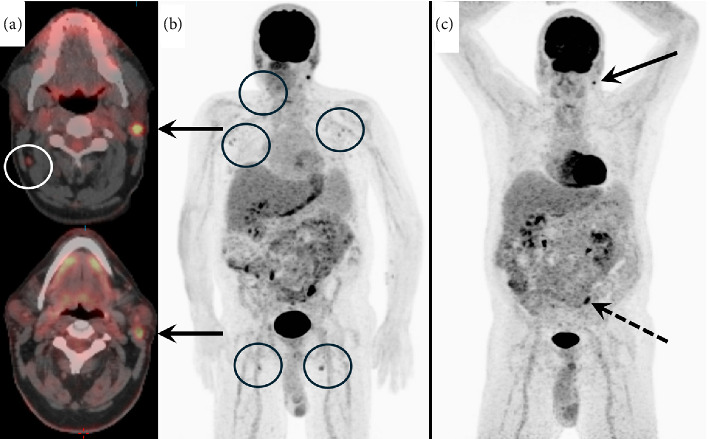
Pre- and postrituximab therapy images. Patient in (a) axial PET CT images of the neck prior to therapy (top) and post-therapy (bottom). In the pretherapy image, a right Level 2 lymph node (white circle) demonstrated pathological uptake. In (b), maximal intensity projection (MIP) image of the FDG PET data demonstrates abnormal nodal uptake above and below the diaphragm (black circles). In (c), post-therapy MIP image are shown with resolution of nodal uptake. Persistent uptake in the left parotid gland (black arrow) and the left abdomen/colon (dashed arrow) is shown. The left parotid is consistent with Warthin's tumor, and the colonic uptake is an adenoma confirmed by colonoscopy.
